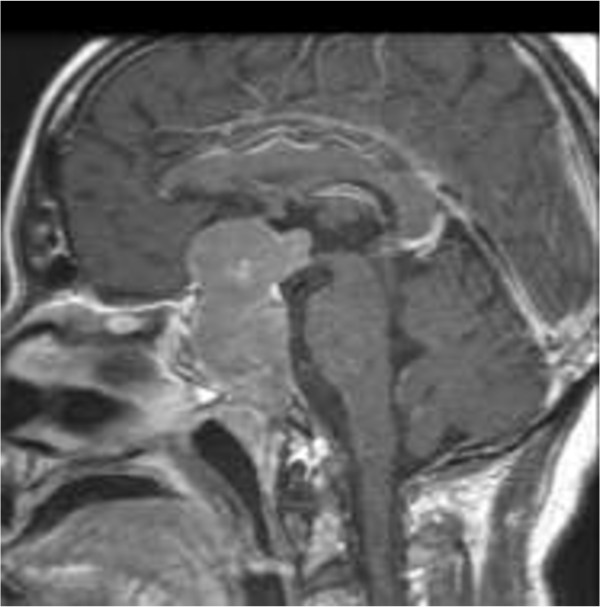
Sagittal Gadolinium-enhanced MRI of the large and invasive macroadenoma, with supra- and infra-sellar extension.
